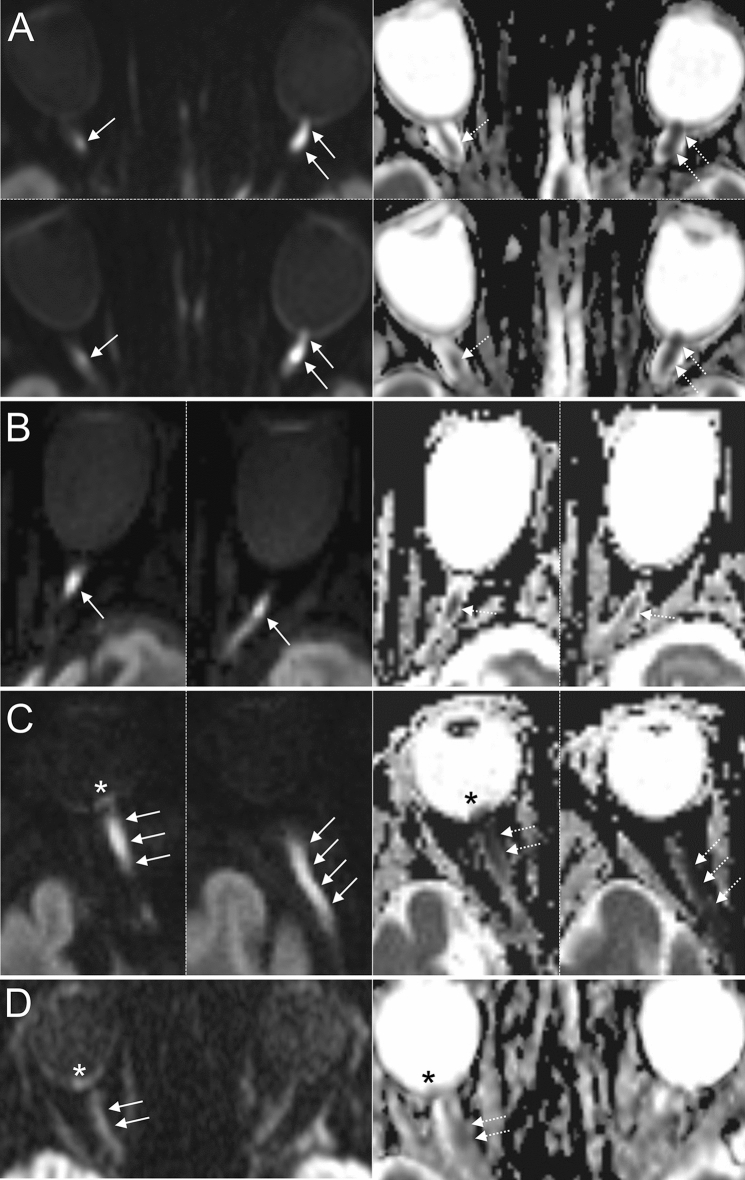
Examples of DWI-MRI in 4 patients with GCA and arteritic ION involving the intraorbital part of the ON (left row: DWI b = 1000 s/mm2, right row: corresponding ADC images). (A) Two consecutive DWI-MRI slices of a GCA patient showing bilateral restricted diffusion of the ON (white arrows; right: intraorbital, left: bordering ONH and intraorbital portion) and corresponding ADC reduction (dotted arrows) suggestive of right posterior ION and left anterior ION. (B) Restricted diffusion of the intraorbital part of the ON on two consecutive DWI-MRI slices (white arrows) and corresponding ADC reduction (dotted arrows) in a patient with left ION. (C) Acute ocular ischemic syndrome in GCA: Two consecutive DWI-MRI slices showing extensive restricted diffusion of the ON involving the ONH and the intraorbital portion (white arrows) with corresponding qualitative ADC reduction (dotted arrows). Note the presence of retinal diffusion restriction indicating concomitant retinal ischemia (asterisks). The findings are indicative of combined arteritic anterior/posterior ION and retinal artery occlusion. (D) Example of 1.5 T DWI showing right-sided restricted diffusion of the intraorbital optic nerve (white arrows) and retina (white asterisk) with subtle qualitative ADC reduction. Abbreviations: ION, ischemic optic neuropathy; DWI-MRI, diffusion-weighted magnetic resonance imaging; GCA, giant cell arteritis; ON, optic nerve; ONH, optic nerve head.
